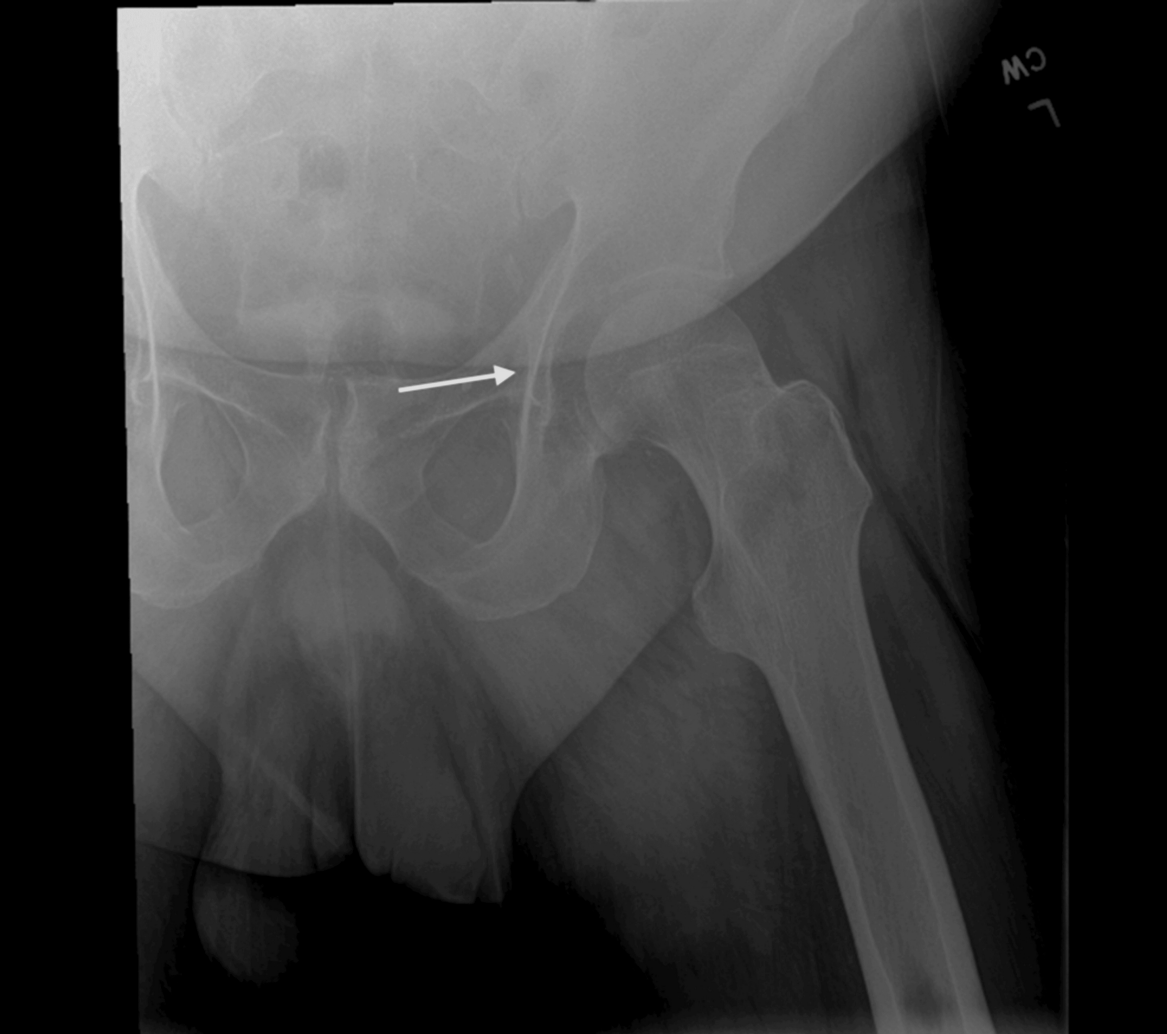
Lateral X-ray of the hip nine months prior to seeking orthopedic evaluation
